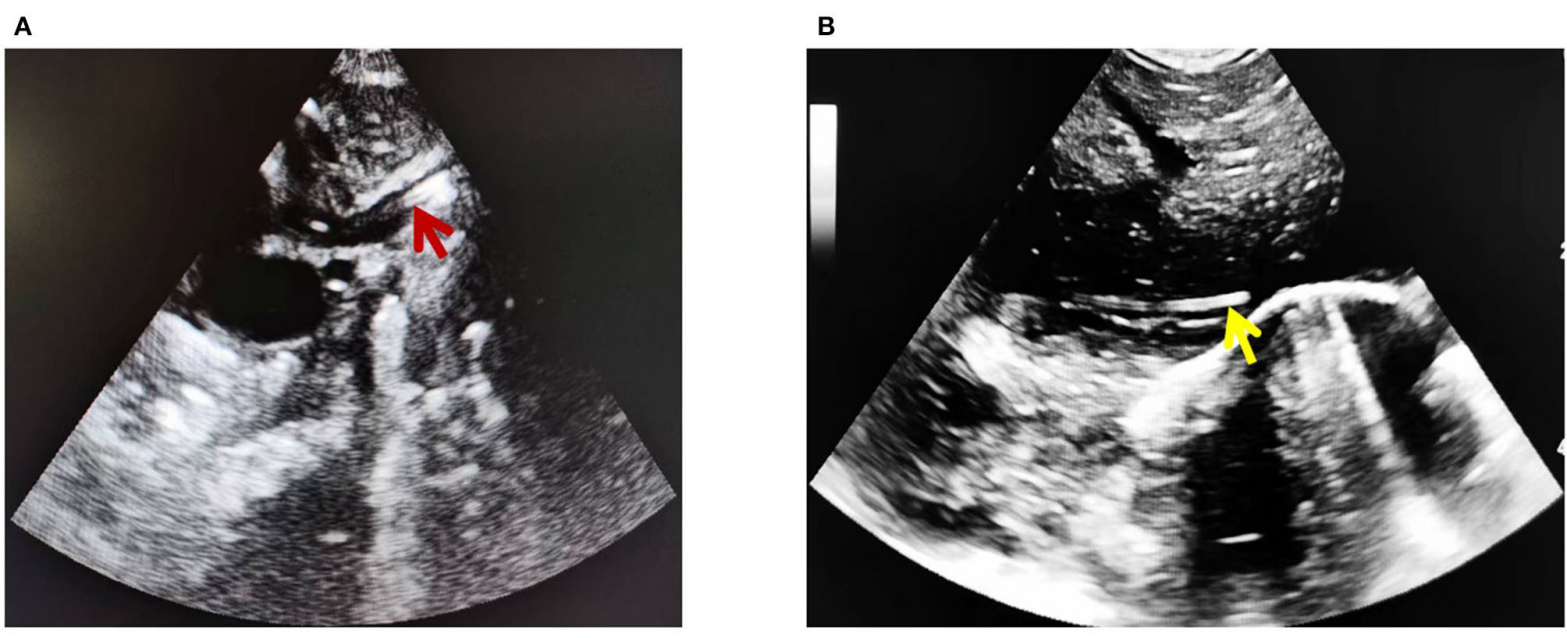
PICC ultrasonic imaging of vena cava. (A) Superior vena cava (red arrow). (B) Inferior vena cava (yellow arrow).
